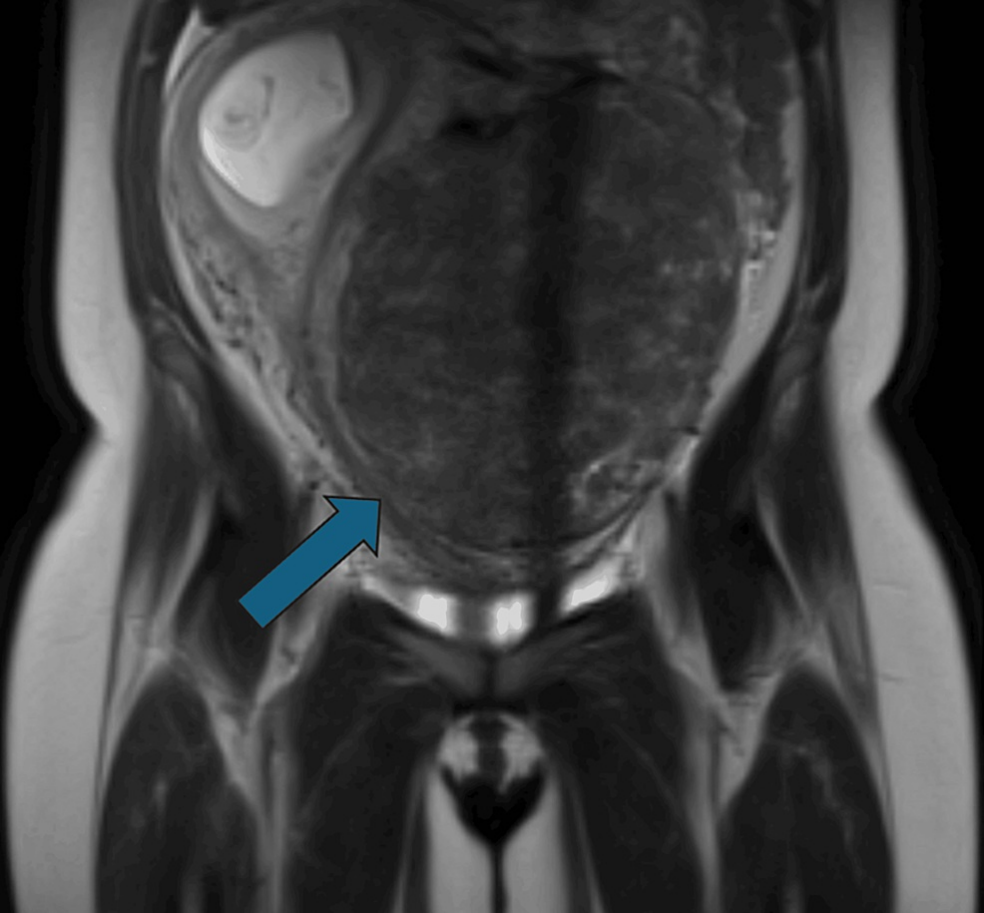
Anterior view of the MRI scan (abdomen and pelvis). The fibroid is seen.
